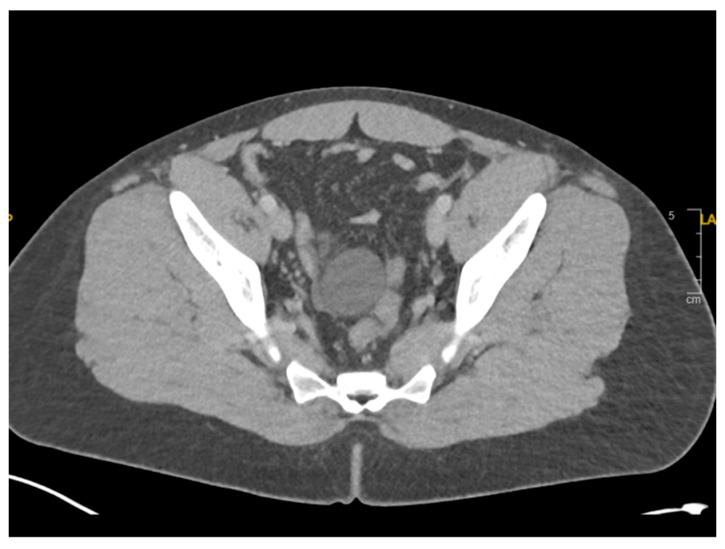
CT abdomen/pelvis in the axial view showed a 4.2 cm cyst in the pelvis, which was not the point of obstruction.
